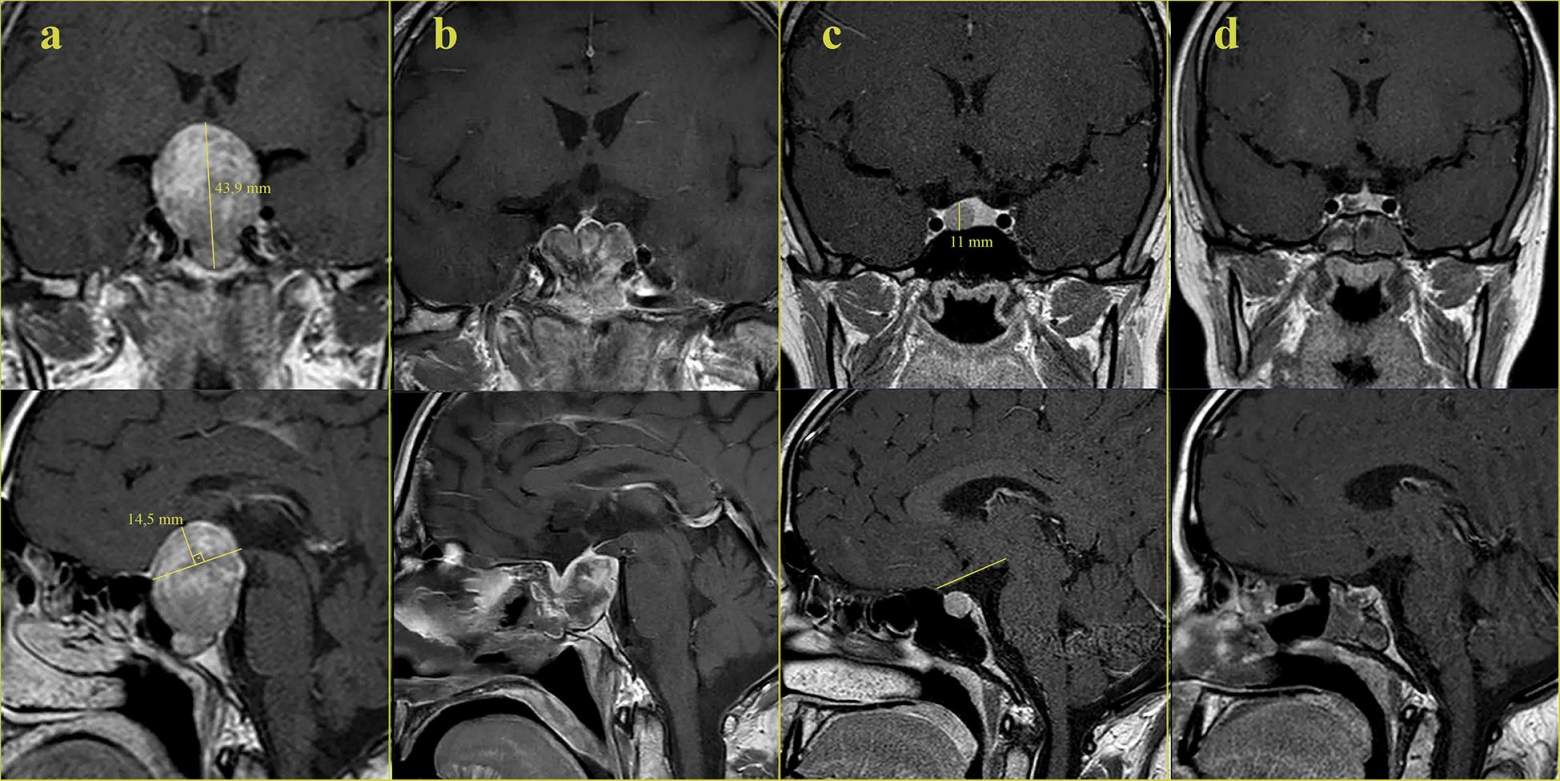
(a) Preoperative MRI views and tumor measurements of a patient with chiasmal distortion. (b) Postoperative MRI views of the same patient with chiasmal distortion. (c) Preoperative MRI views and tumor measurements of a patient without chiasmal distortion. (d) Postoperative MRI views of the same patient without chiasmal distortion.
